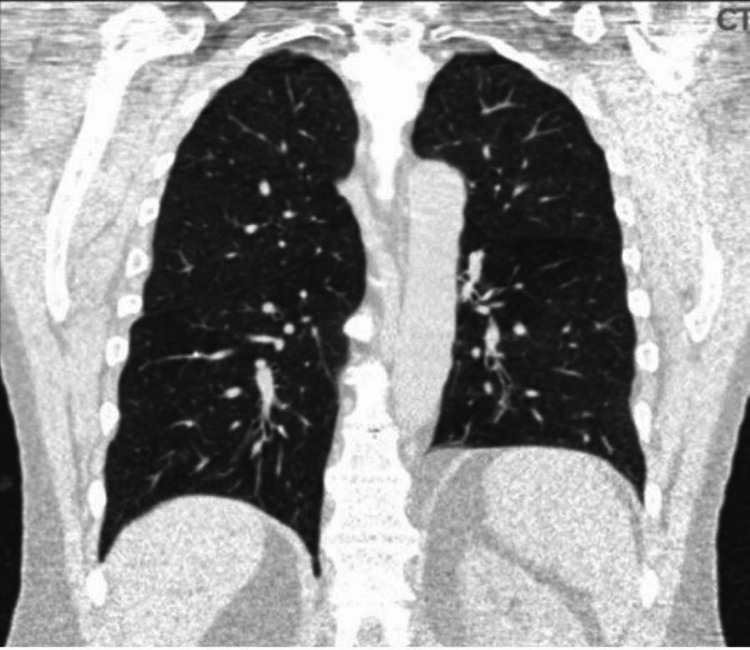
Coronal low-dose CT chest image in Case 4 demonstrating emphysematous changes A coronal low-dose CT chest image obtained before the index pulmonary evaluation demonstrates emphysematous changes, supporting COPD-associated radiographic features in a patient who also demonstrated bronchodilator responsiveness. COPD: chronic obstructive pulmonary disease
